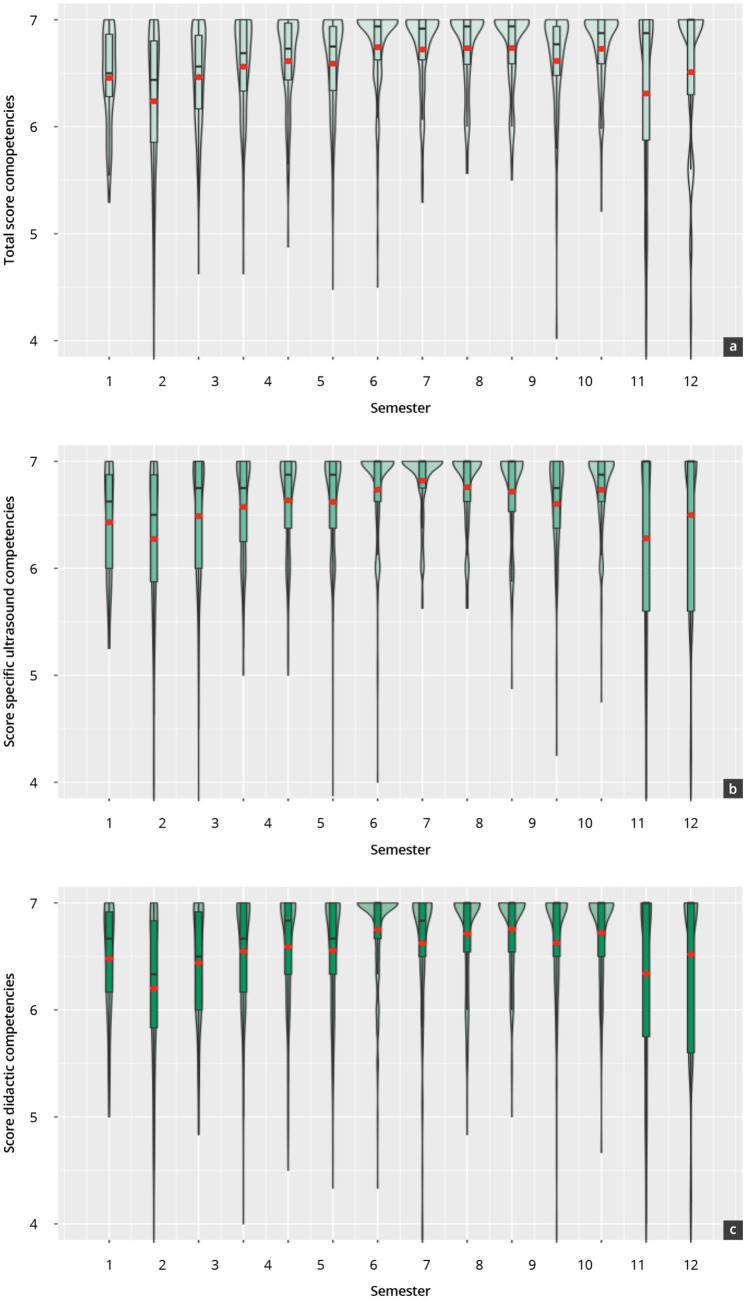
Evaluation results of tutor competencies by course participants from 2017 to 2014 across “total competencies” (A); “specific ultrasound competencies” (B); and “didactic competencies” (C).
